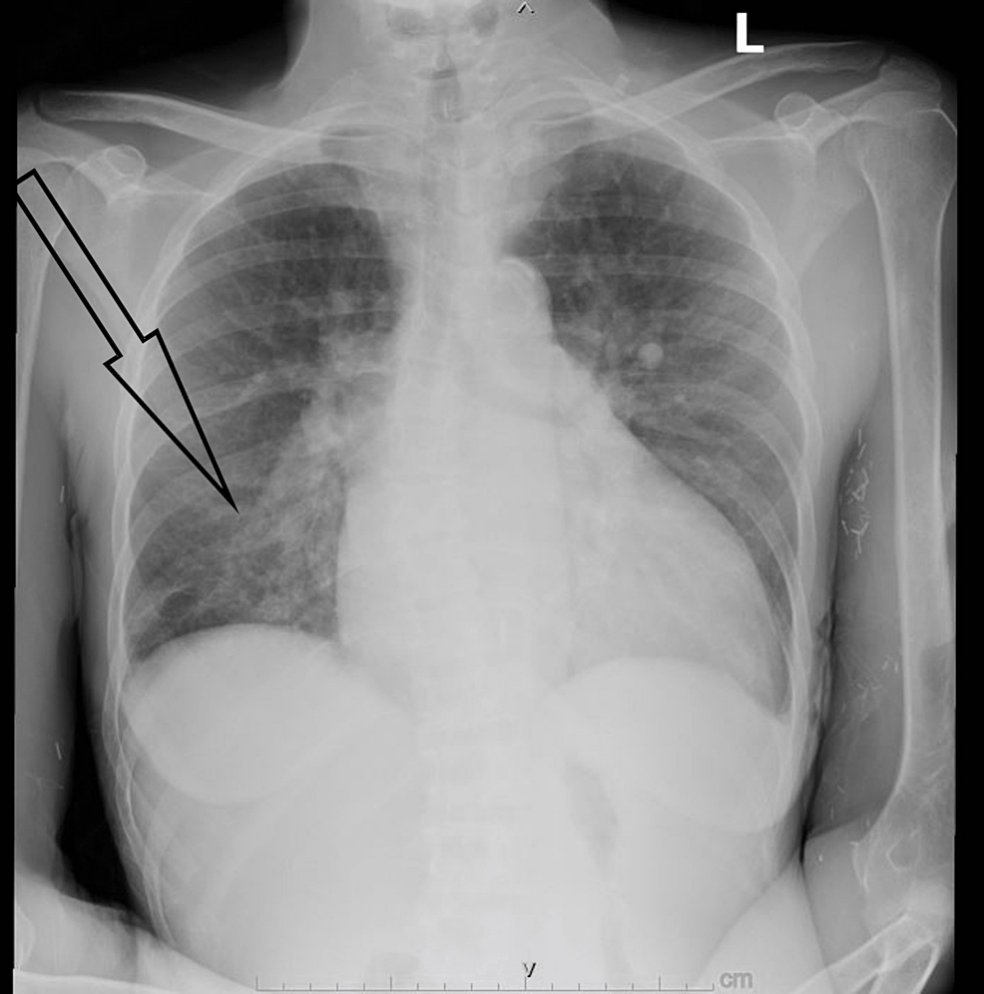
Chest X-ray showing right lower lobe pneumonia
